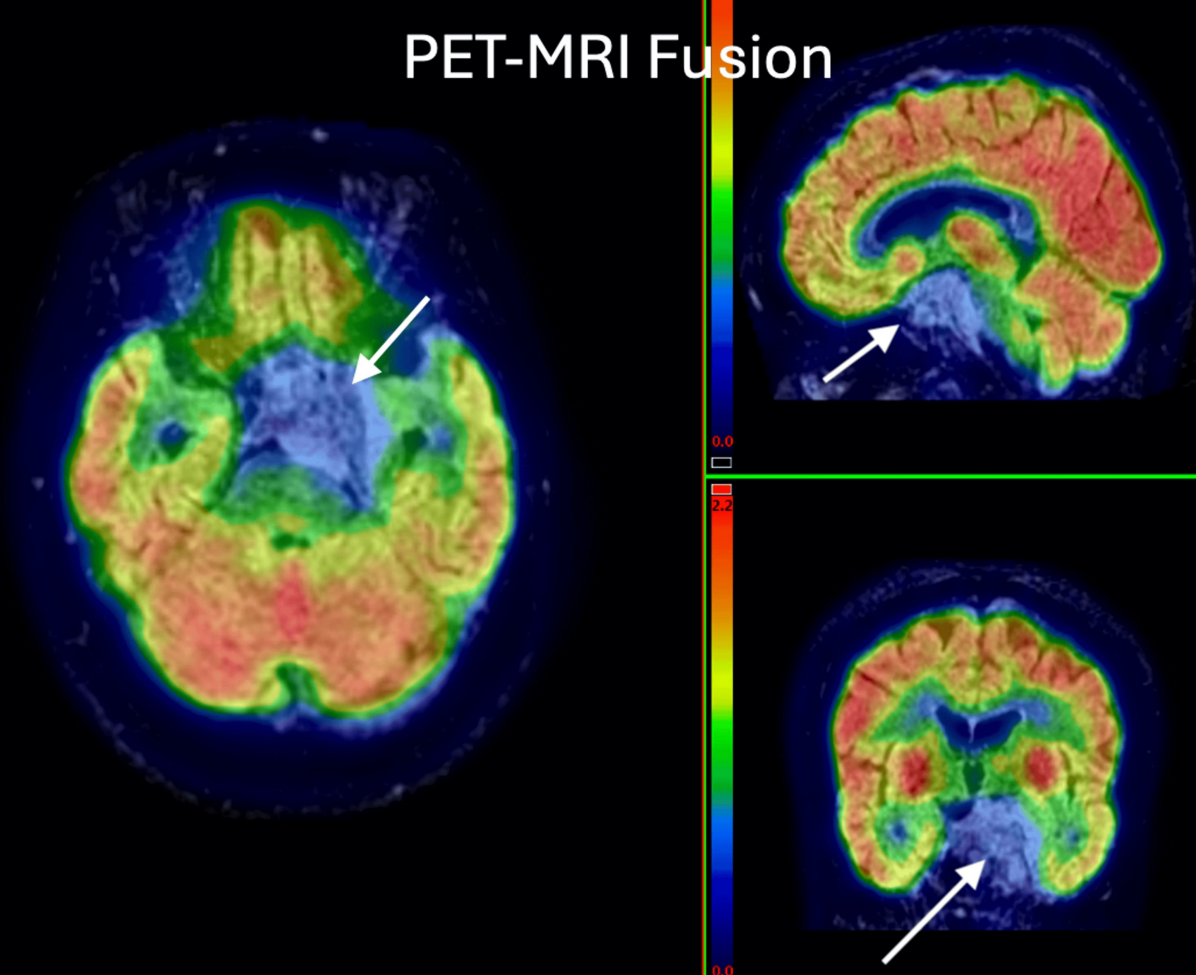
71-year-old female with a history of imbalance while walking and visual disturbance. PET-MRI brain study shows a large pituitary macroadenoma with no FDG activity. PET: positron emission tomography; MRI: magnetic resonance imaging; FDG: fluorodeoxyglucose
